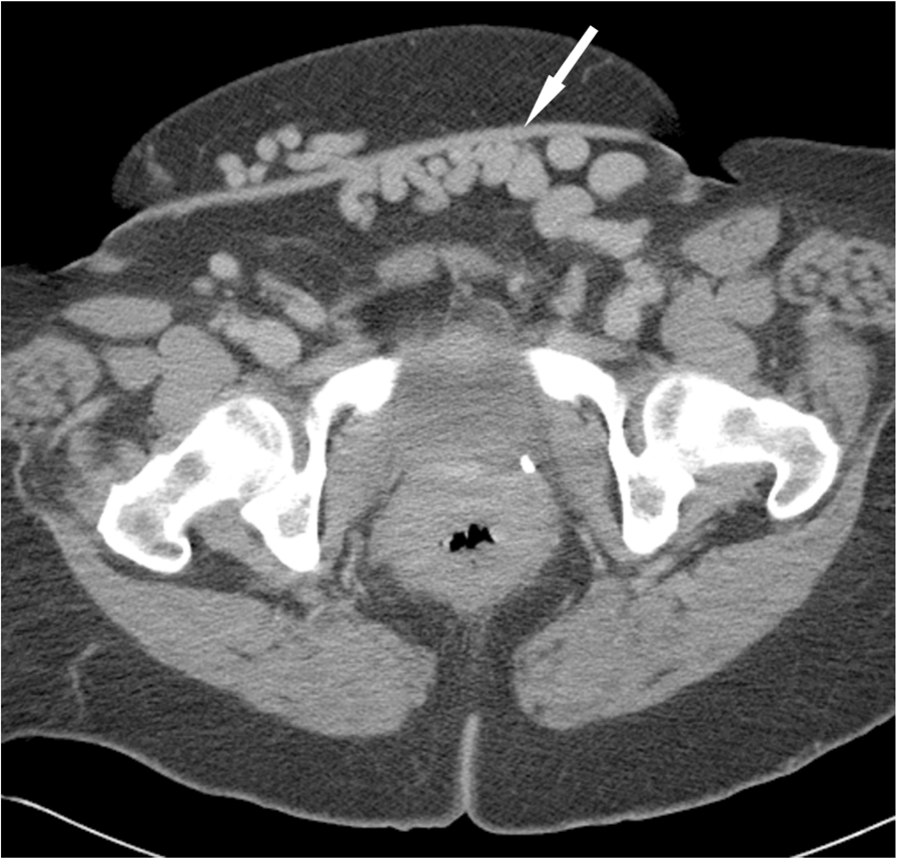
Axial CT of a 55-year old woman presenting with bilateral lower extremity edema and pain. Torturous collaterals connecting the great saphenous veins are noted coursing through the anterior pelvic wall adipose tissue (white arrow)
